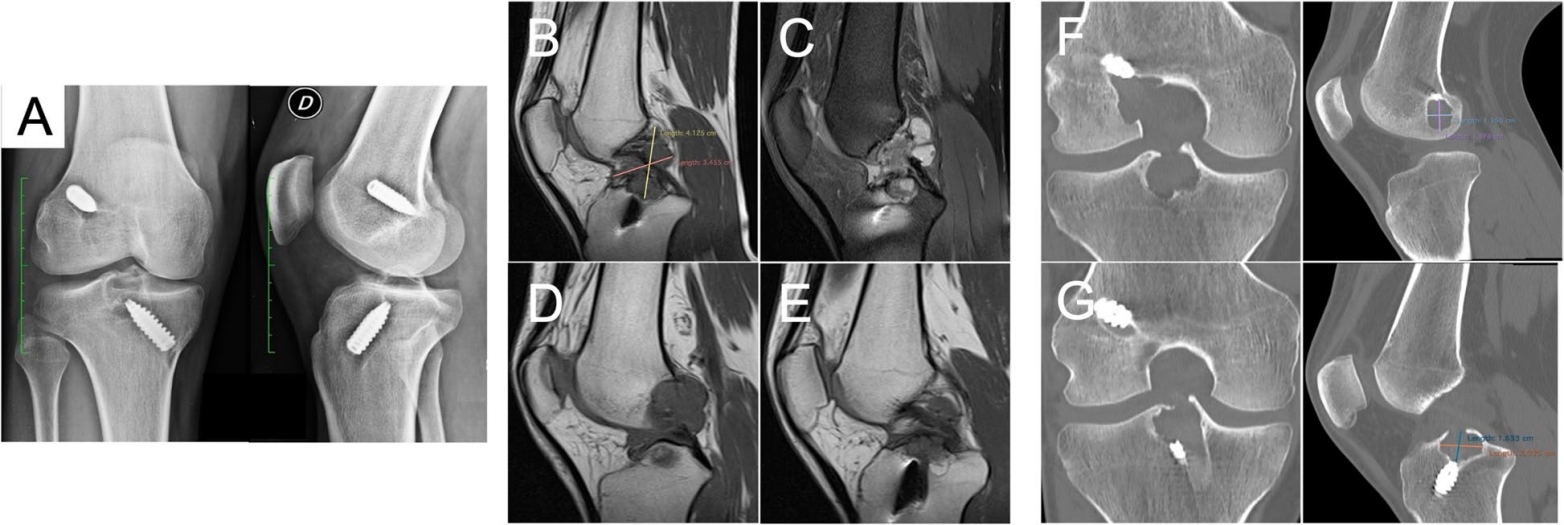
A, preoperative X-ray exam showing tunnel enlargement and osteolytic resorption of the intercondylar eminence. B-E, MRI representative images showing the size of the pseudo-myxoid mass occupying the intercondylar notch (B). STIR images demonstrated the presence of concamerated fluid cysts within the mass with no clear signs of the ACL graft (C). The lesion expanded both towards the femoral (D) and tibial tunnels (E). F-G, CT representative images showing the morphology and maximum diameter of the femoral (F) and tibial (G) osteolytic cavities surrounding the tunnels
